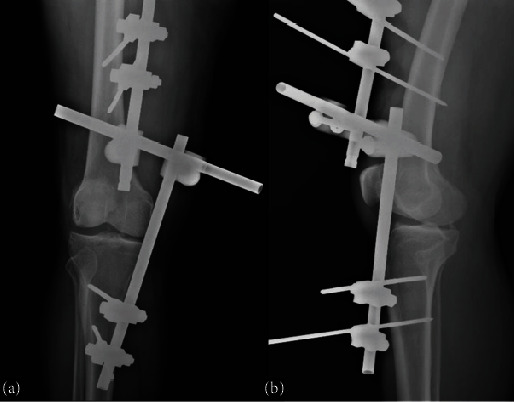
X-ray: (a) AP and (b) lateral of the right knee post external fixation.
